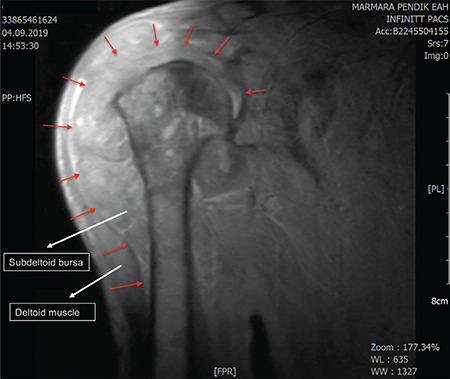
Significant thickening of the subdeltoid bursa was evident upon shoulder magnetic resonance imaging.
